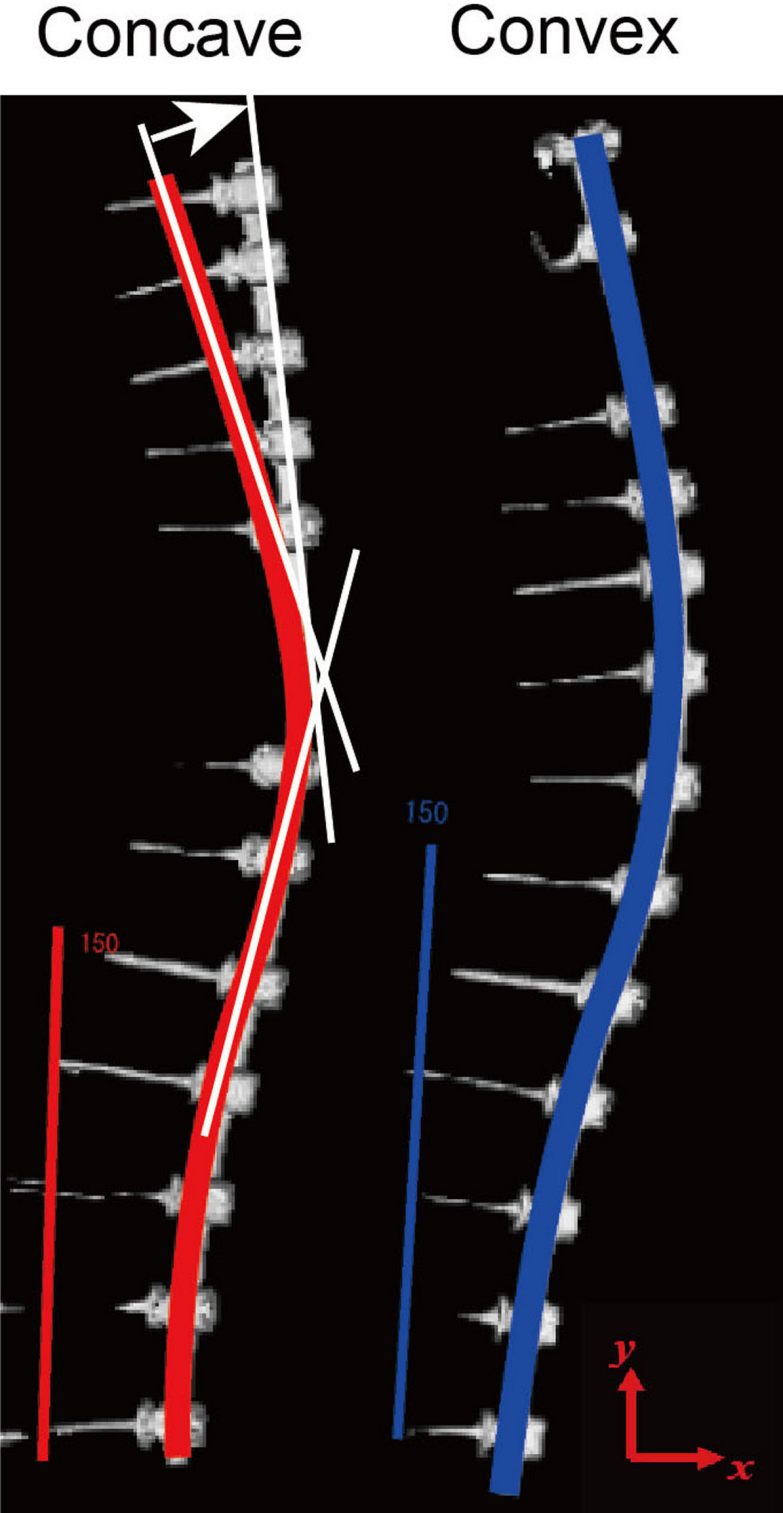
Collection of rod geometry. The rod geometry was collected from intraoperative tracing and postoperative 3D-CT images. Rod contour decreased in postoperative 3D-CT (arrow).
